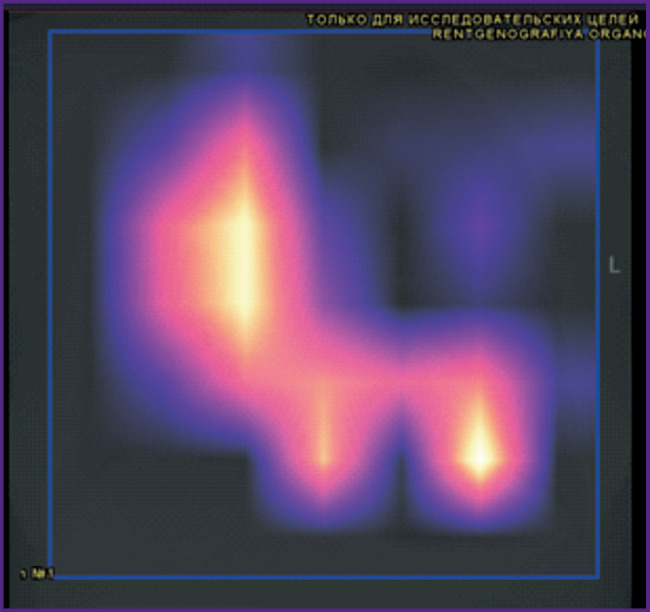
An example of SaMD-AI technical flaws (1.2 and 2.8, see the Table) using evidence from chest radiography
